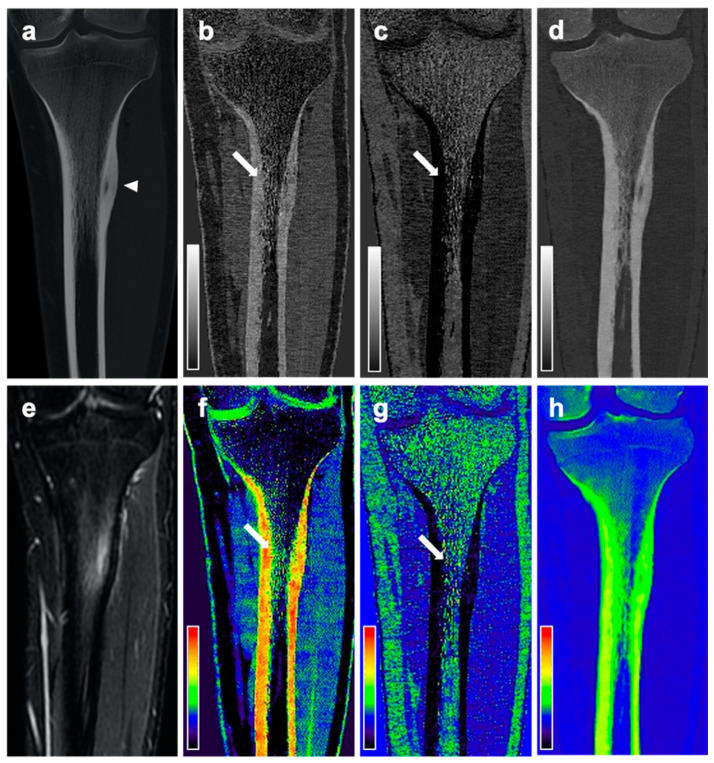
CT and MR images of a 19-year-old male patient with intracortical OO in the left tibia diaphysis. Coronal reformation of the conventional CT examination (a) reveals the nidus in the left tibia diaphysis (arrowhead). Material-specific volume fraction maps for red marrow/water (b), yellow marrow/fat (c), and hydroxyapatite (d), and respective color-coded overlays (f–h) reveal an increase in water-specific volume fraction in the red-marrow map (b,f; arrow) and a decrease in fat-specific volume fraction in the yellow-marrow map (c,g; arrow). The corresponding coronal STIR MR image confirms the presence of an edema-equivalent signal alteration in the left tibia diaphysis (e).
